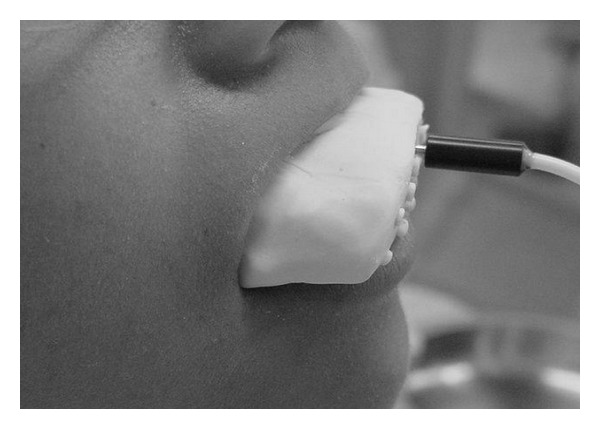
Laser Doppler probe stabilized by a silicone splint, applied in one volunteer.
